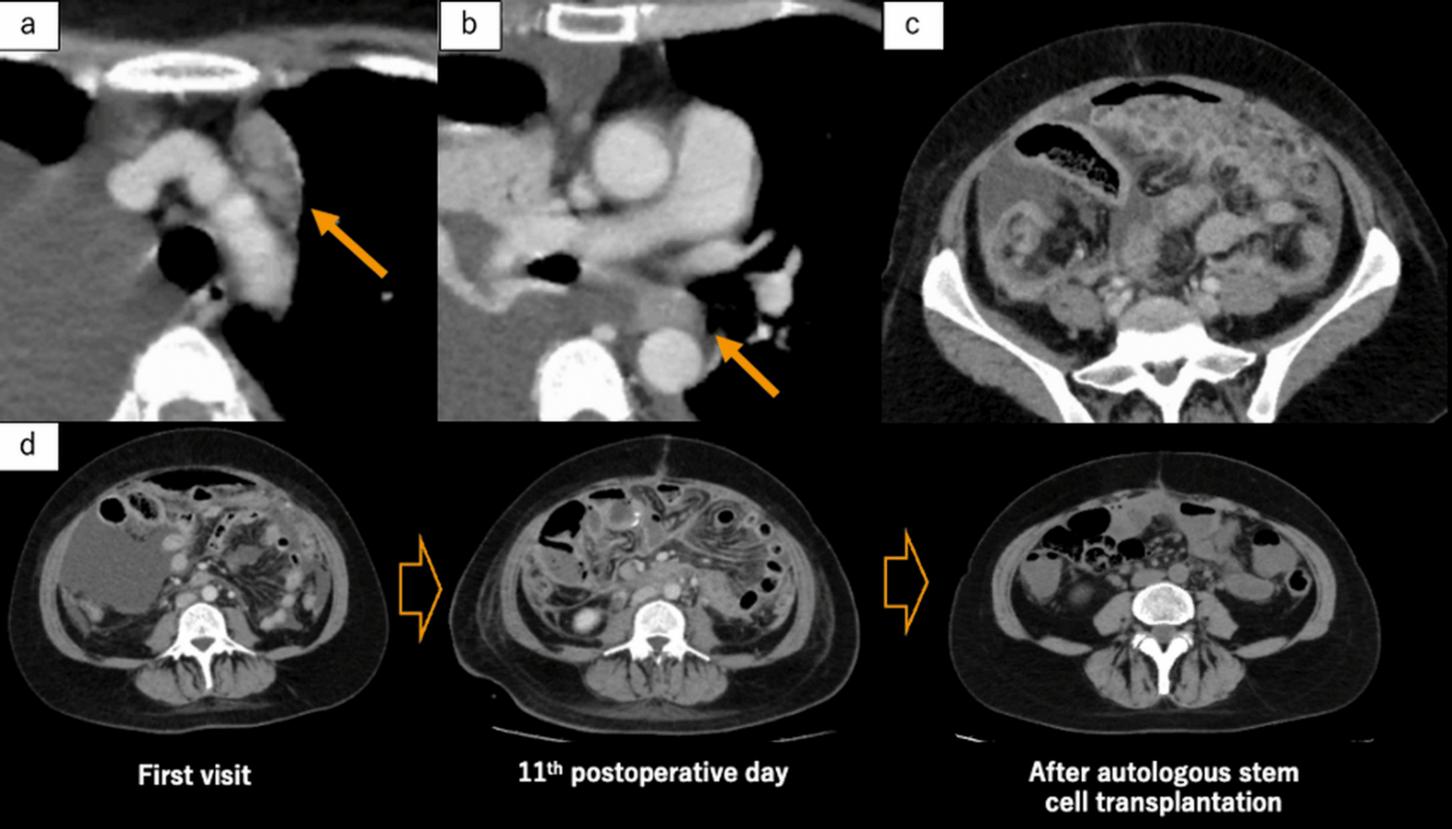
Follow-up contrast-enhanced CT scan on postoperative day 11 (a) Enlargement of the thymic or anterior mediastinal lymph nodes. (b) Enlargement of the lymph nodes below the tracheal carina. (c) Diffuse soft tissue proliferation in the abdominal cavity. (d) The soft tissue proliferation along the mesentery observed on the CT scan at the time of the first emergency department visit worsened on postoperative day 11 but markedly improved following autologous stem cell transplantation.
